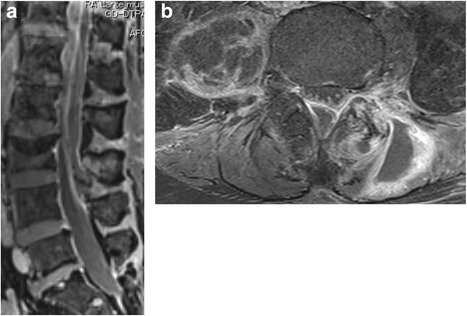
T1-weighted MRI with gadolinium enhancement at the L3/4 level. Two weeks after lumbago onset, the mass (enhanced at the edge) had spread widely to iliopsoas muscles, paraspinal muscles, and the epidural space. a Sagittal view. b Axial view
